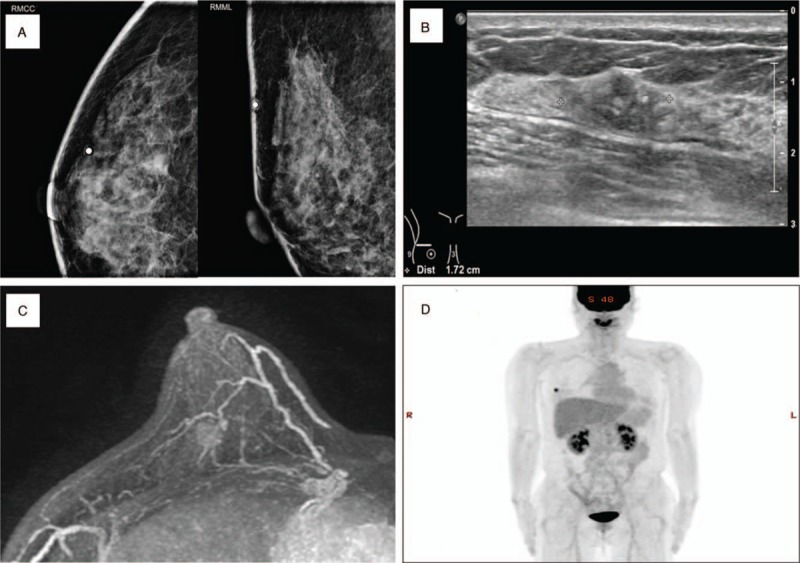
Mammography (A), breast ultrasonography (B), breast magnetic resonance imaging (MRI) (C) and positron emission tomography-computed tomography (PET-CT) (D) findings showing irregular enhancing mass in the right breast without axillary lymph node metastasis. There was no evidence of distant metastasis.
